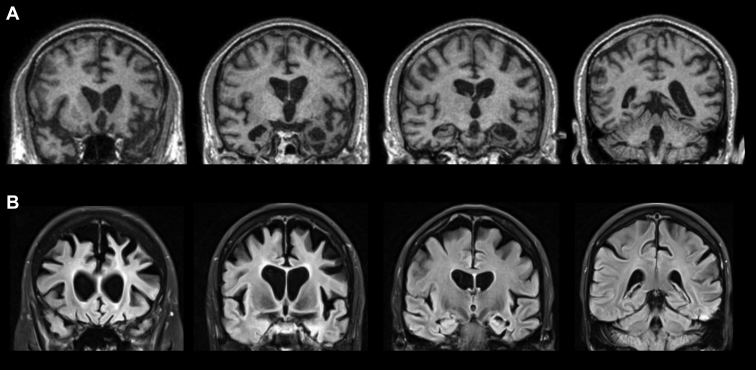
Magnetic resonance imaging of case 1 (A) and case 2 (B) showing coronal volumetric T1 (case 1) and FLAIR (case 2) scans. Asymmetrical, left more than right, temporal greater than frontal atrophy is seen in case 1, whereas in case 2, there is relatively symmetrical bilateral frontal more than temporal lobe atrophy.
